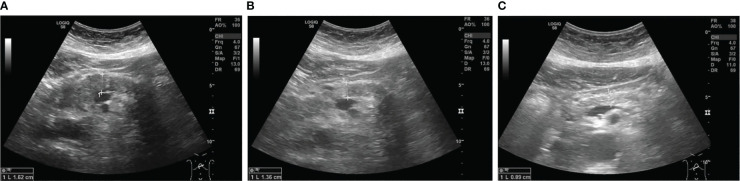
Sonographic images of the pancreatic body showing diffuse hypoechoic swelling upon admission (A), which decreased under steroid treatment (B) and 11 months after the first dose of vedolizumab (C).
